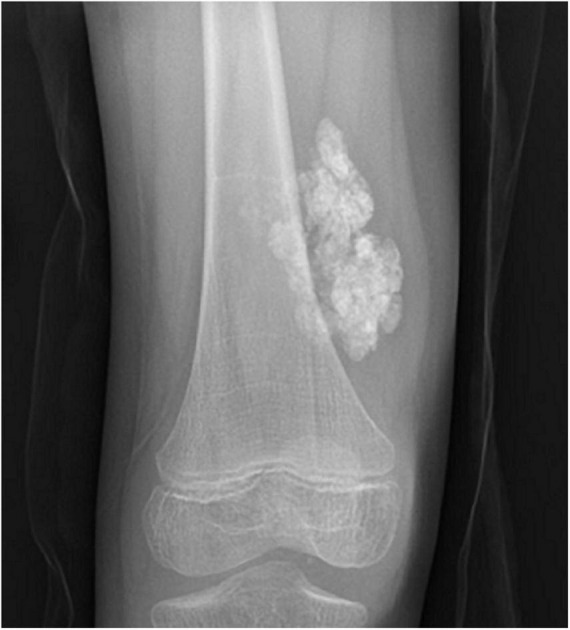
Plain radiograph of calcinosis lesions in the subcutaneous tissues adjacent to the right knee of a child with juvenile dermatomyositis. Permission obtained from the patient for publication of this image.
